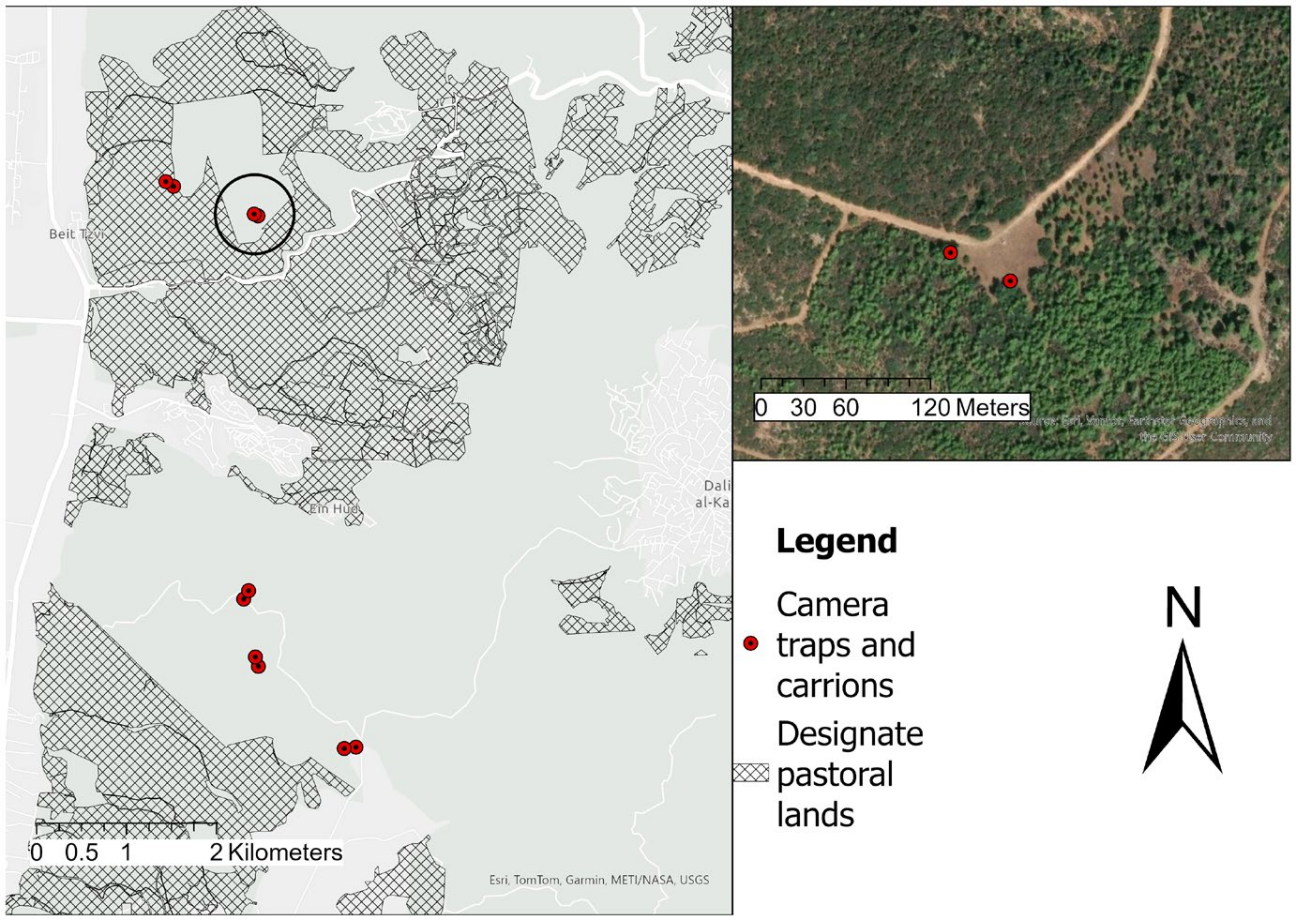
Crmel National Park study site and sampling units. The upper right panel shows a single sampling unit composed of carrion+CT (red dots).
